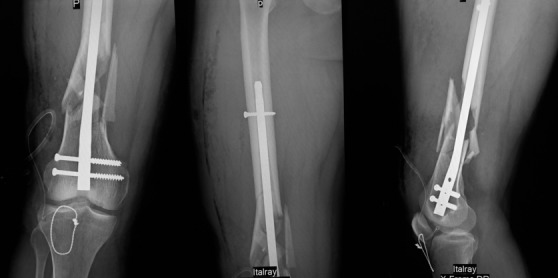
radiographies de contrôle en post opératoire de face et de profil à 30° de flexion avec un indice de Caton et Descamps à 1,1
